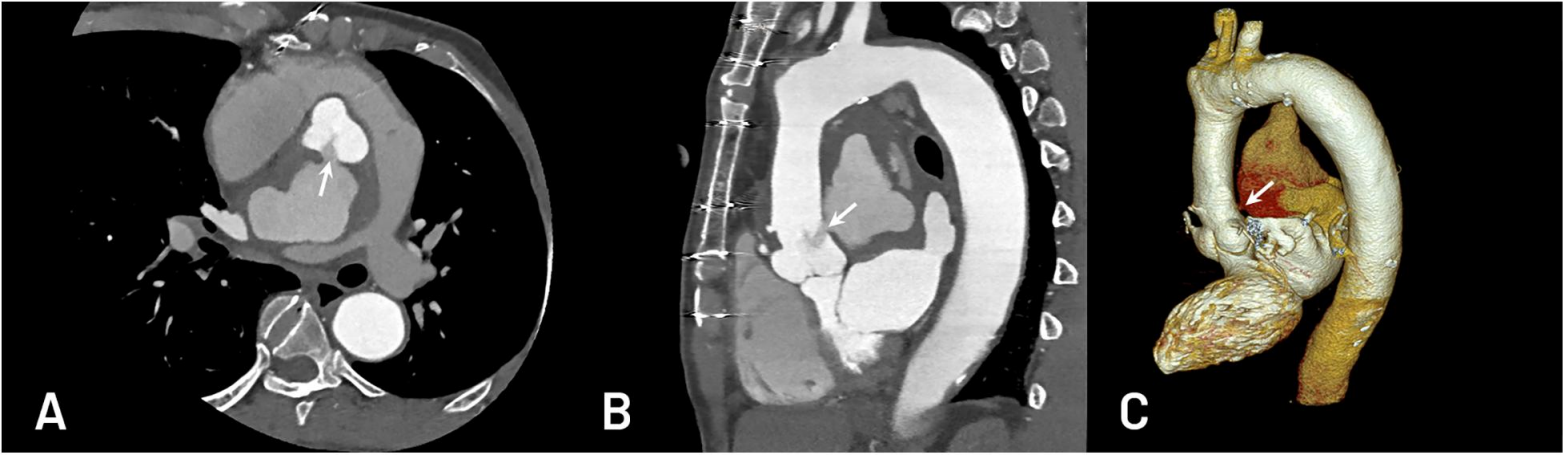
CTA showed a giant posterior ascending aortic pseudoaneurysm with a 7 mm neck (A) above the non-coronary cusp and partially compressed the left atrium and right pulmonary artery (A,B). 3D reconstruction of pseudoaneurysm (C).
